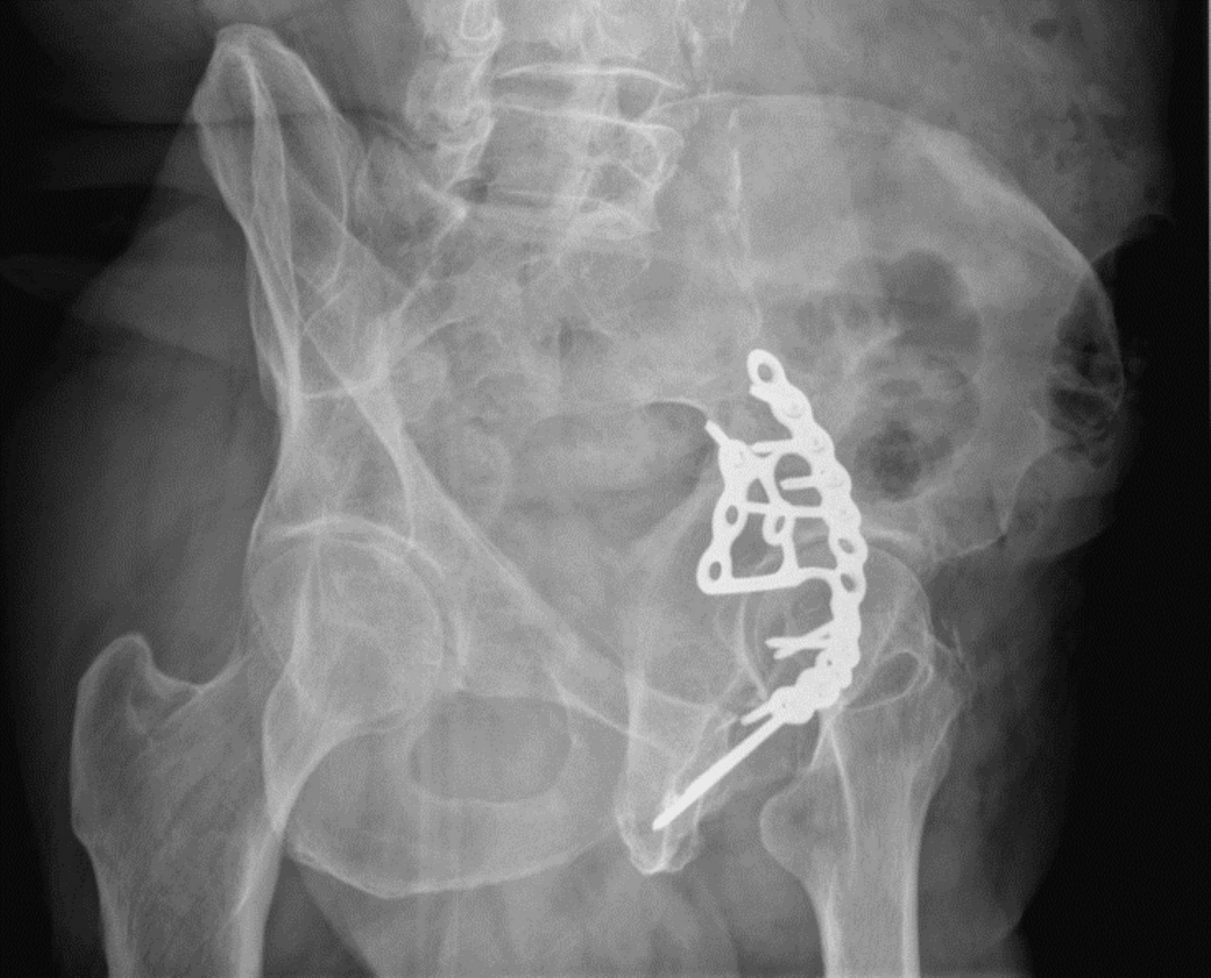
Iliac oblique radiograph of the left hip in a patient, five years following fixation of a displaced acetabular fracture using an anatomical suprapectineal plate
